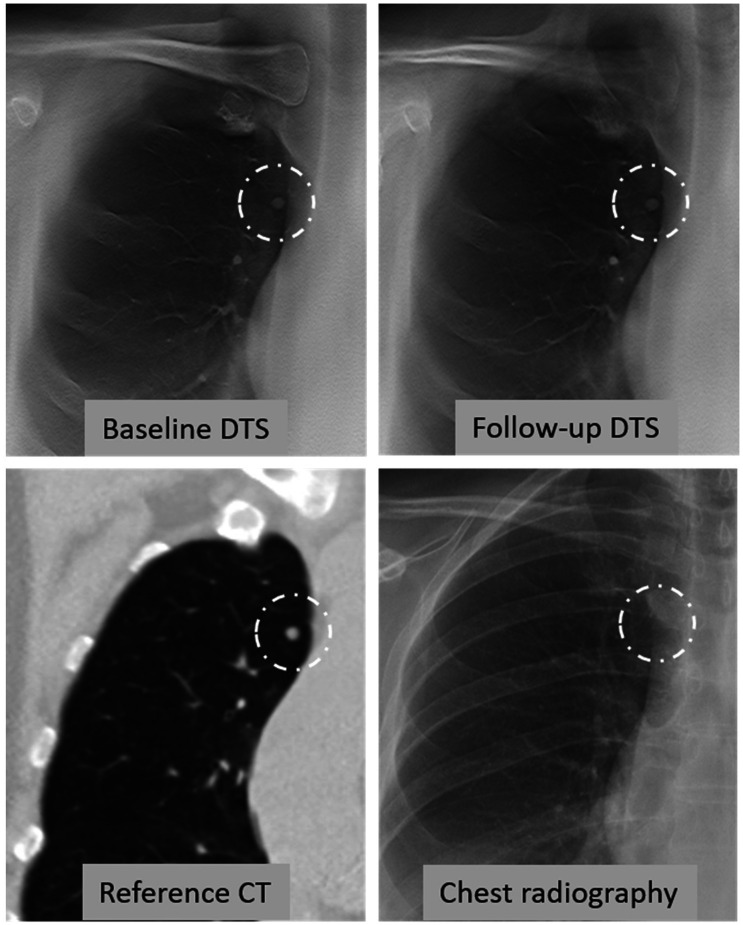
Example of a well-depicted nodule on DTS. A 65-year-old woman, previous smoker (3 pack-years), no history of cancer. Incidental, solid nodule in the right upper lobe with an average diameter of 6 mm on baseline and follow-up CT. Volumetric CT estimates were 66 mm^3^ on baseline, and 87 mm^3^ on follow-up, with a resulting volumetric increase of 32% and VDT of 1300 days. Average diameter based on two diameters on DTS was 5 mm for both baseline and follow-up for both observers, and calculated volume based on the same measurements were 52 mm^3^ and 65 mm^3^ (baseline and follow-up observer 1), and 63 mm^3^ and 71 mm^3^ (baseline and follow-up observer 2), resulting in an volumetric increase of 25% and 13%. VDT was 1500 and 2800 days for observers 1 and 2, respectively. The nodule is not clearly visible on chest radiography. CT, computed tomography; DTS, digital tomosynthesis; VDT, volume doubling time.
